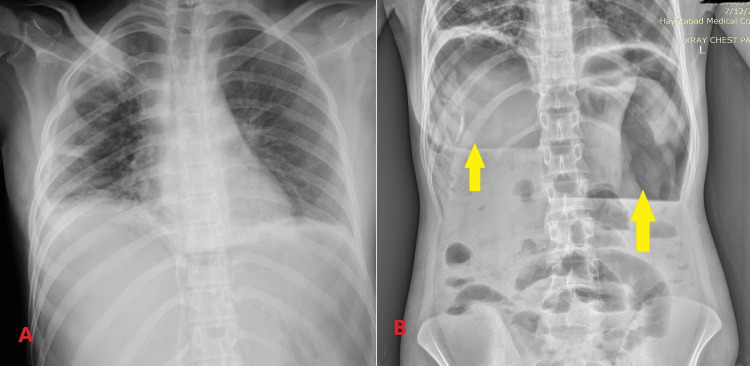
(A) X-ray on arrival shows no abnormality. (B) X-ray of the abdomen on the second day of admission shows a huge collection of air under the bilateral diaphragm (shown by yellow arrows)
